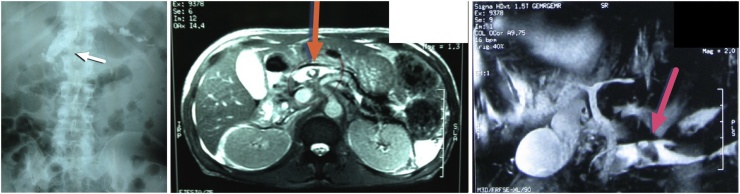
Imaging showing opacities: a. Plain abdominal X-ray in the region of middle and right upper quadrant abdomen; b. MSCT and c. MRCP is showing opacities in pancreatic duct and distal common bile duct.
